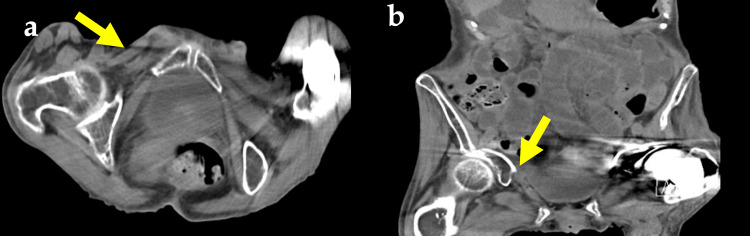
Abdominal computed tomography scan showing obturator hernia reduction (arrows): (a) axial view; (b) coronal view.
